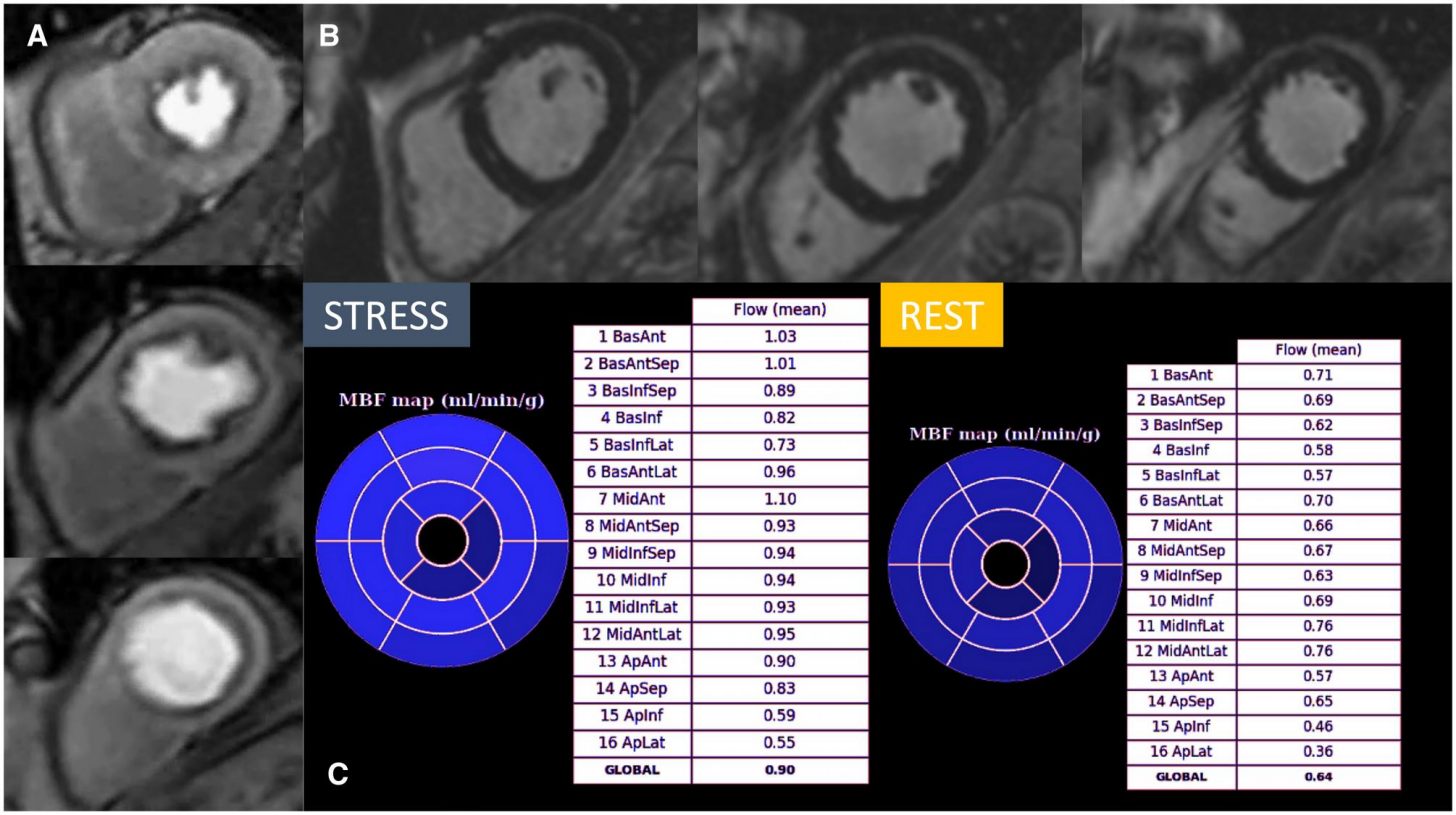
Cardiac MR images of a patient with coronary microvascular dysfunction (MVD): Short-axis stress perfusion images (A) reveal diffuse inducible subendocardial perfusion defects across various vascular territories, whereas late gadolinium enhancement (LGE) images (B) show no visible scar. In some cases, patients with MVD may present without visually apparent subendocardial perfusion defects; however, quantitative perfusion analysis (C) may reveal poor hyperemic blood flow, with a significantly reduced myocardial perfusion reserve (MPR), which can be calculated by dividing stress myocardial blood flow (MBF) by rest MBF.
